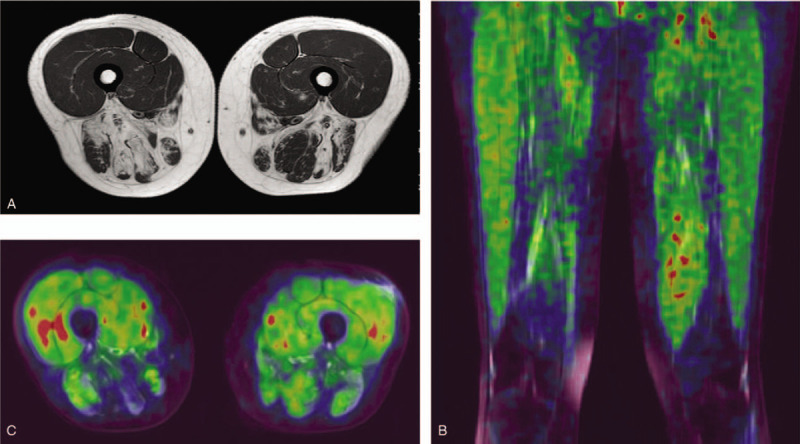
MRI & PET images. (A) MRI image showed atrophy and partial fatty transformation of bilateral lower limb muscles. (B) Tau PET images showed elevated radioactive uptake in the bilateral quadriceps. (C) PET axial image revealed the SUV levels of quadriceps = 0.73/0.67 (right/left).
